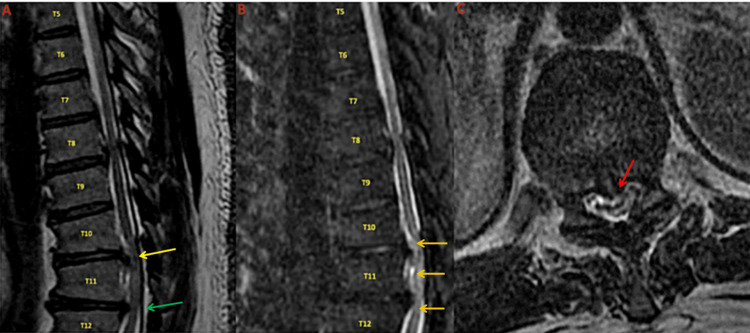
MRI thoracic spine A. MRI T2 weighted image in the sagittal plane showing multilevel herniated discs. The largest herniated discs and the ones responsible for the patient's acute symptoms are at T10-T11 (yellow arrow) and T11-T12 (green arrow). B. MRI STIR sequence in the sagittal plane demonstrating multiple disc herniations. The spinal cord is hyperintense from T10-T12 compatible with edema or ischemia in the acute setting (yellow arrows). C. MRI T2 weighted image in the axial plane at the level of T10-T11, demonstrating a large posterior central disc protrusion causing signiﬁcant cord compression (red arrow). MRI: Magnetic resonance imaging; STIR: Short T1 inversion recovery; T: thoracic
